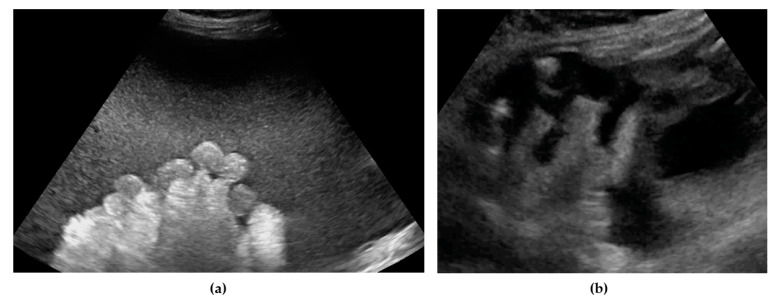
Ultrasound images of small bowel mesentery: (a) involvement of mesentery root (mesenteries adhere to each other); (b) no involvement of mesentery root (separation of mesenteries). Note: the best visualization in dynamic examination (see Video S2).
